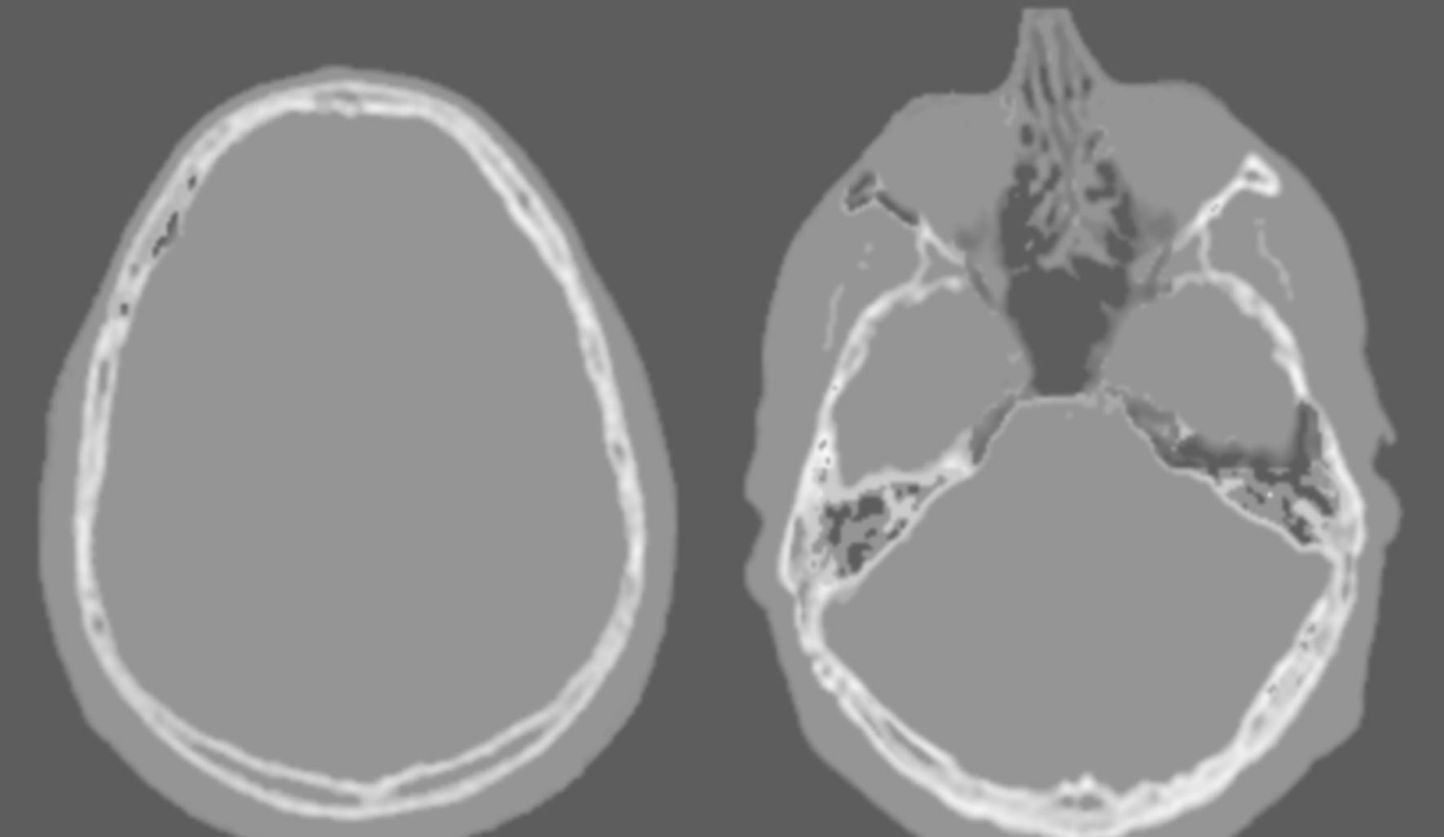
Examples of post-processed pseudo—CT images created from ZTE imaging
